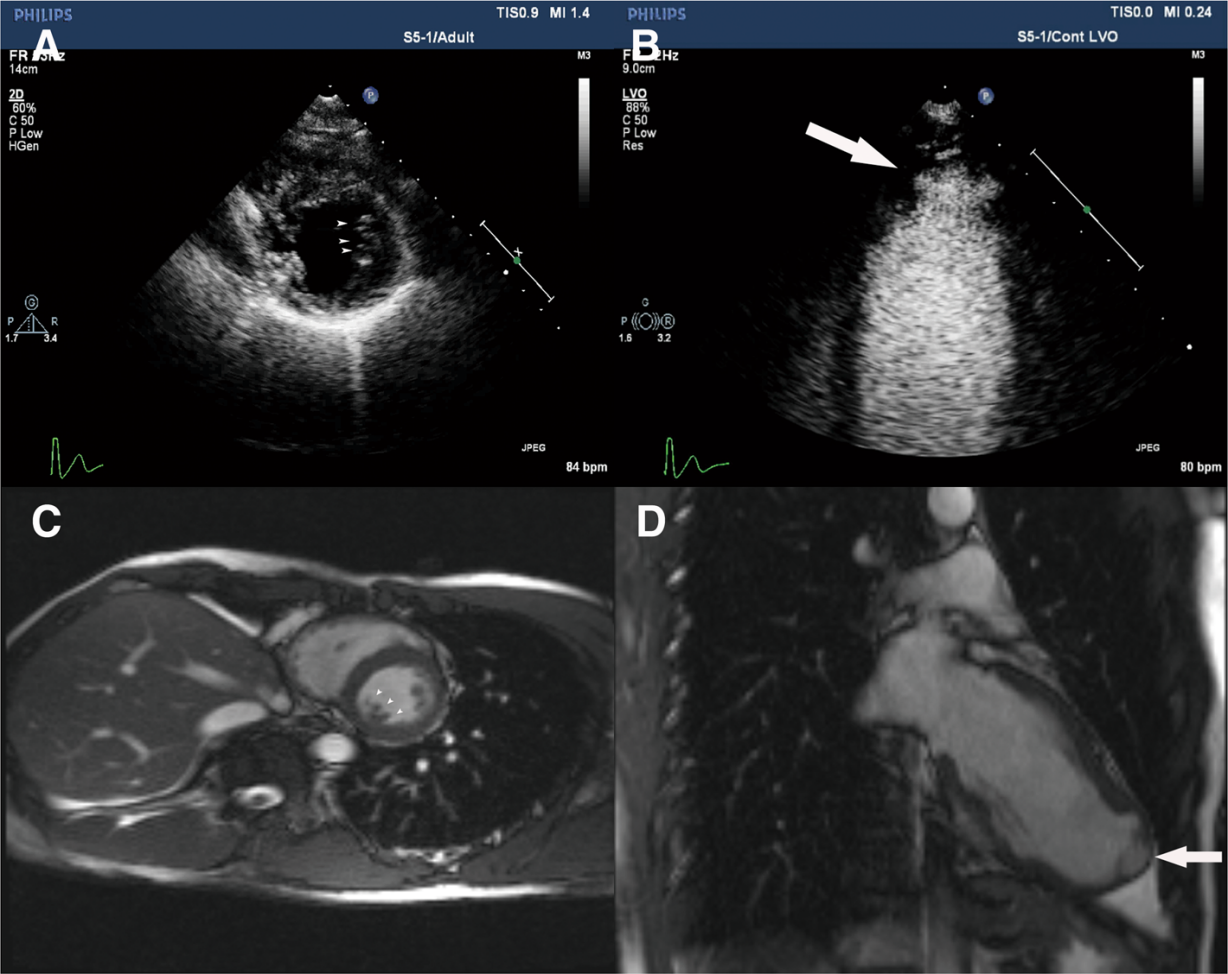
Contrast echocardiography and cardiac magnetic resonance at diagnosis. Panel a, b Contrast echocardiography. a Apical short-axis view of left ventricle showing prominent left ventricular trabeculae and deep intertrabecular recesses (*arrowheads*). b Transapical view of the left ventricular apex showing an aneurysm-like out-pouching structure with a wide connection to the left ventricle (*arrow*). Panel c, d Magnetic resonance imaging. c Left ventricle short-axis view showing prominent left ventricular trabeculae and deep intertrabecular recesses (*arrowheads*). d Left ventricular outflow tract view showing an apical protrusion with a wide connection to the left ventricle (*arrow*)
